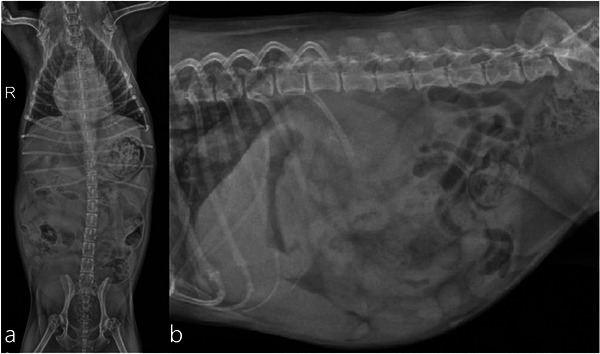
Follow‐up abdominal radiographs after 7 months of therapy. (a) Ventrodorsal view showing no evidence of the previously identified retroperitoneal mass. (b) Right lateral view confirming the absence of a space‐occupying lesion, consistent with complete radiographic remission.
